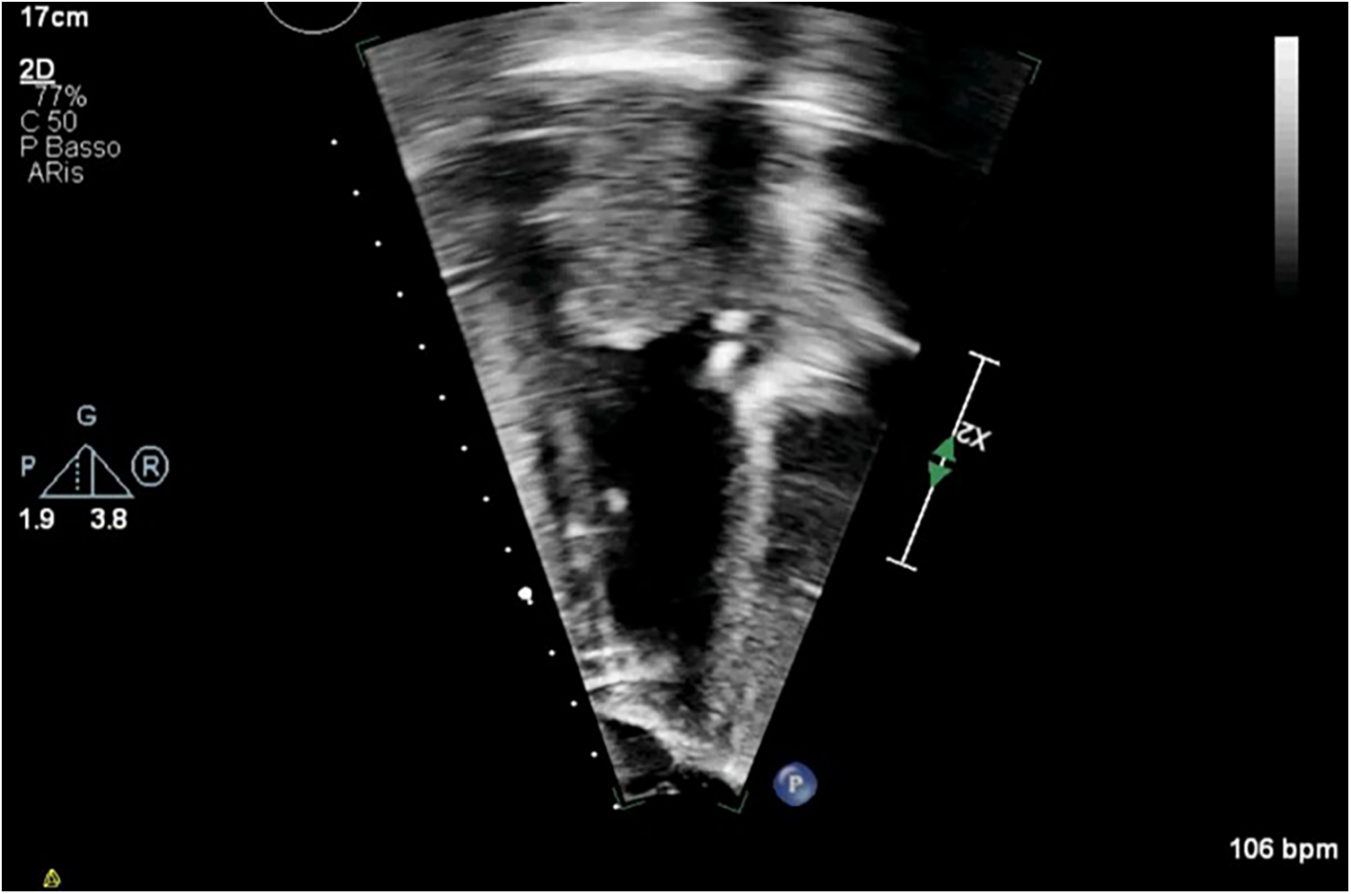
2D apical 4C view showing a huge right retroatrial hematoma after LVAD implantation.
